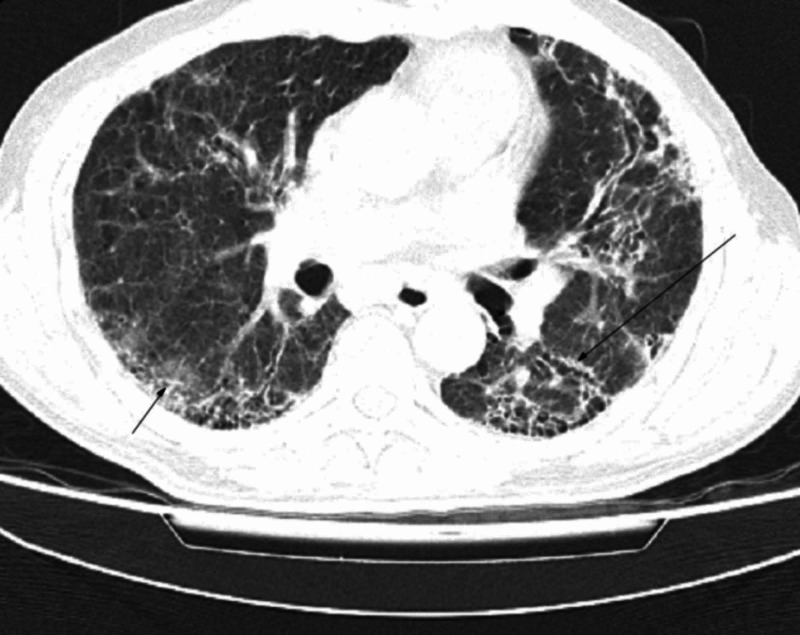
High-resolution computed tomography scan of a COVID-19 patient with preexisting idiopathic pulmonary fibrosis New-onset bilateral ground-glass haze (*black arrows*) superimposed on underlying usual interstitial pneumonia
